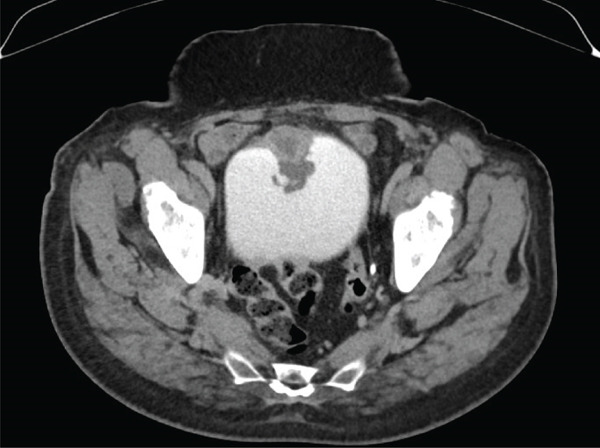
Computed tomography intravenous pyelography study (axial view).
